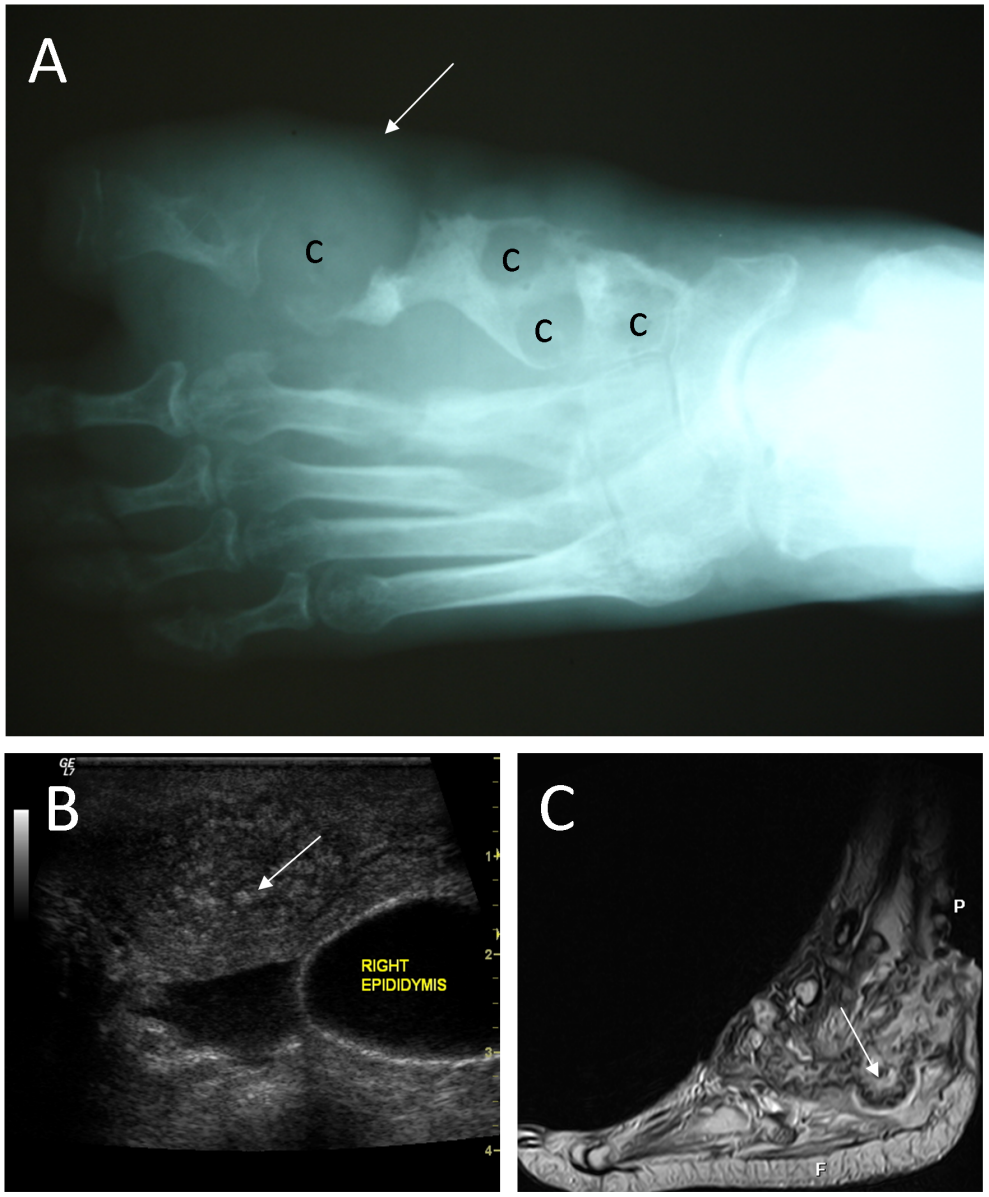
Imaging techniques used in mycetoma. A: A radiograph of the foot, showing soft tissue shadow (arrow), and multiple large cavities (c) in line with eumycetoma. B: A typical sonogram of scrotal eumycetoma. In this sonogram multiple cavities with thick walls and multiple hyper-reflective echoes (arrow) are seen, which are in line with grains. C: An MRI of the foot, showing massive soft tissue and bone destruction. In this MRI, grains appear as conglomerates of small (2–5 mm) round hyperintense lesions (arrow).
